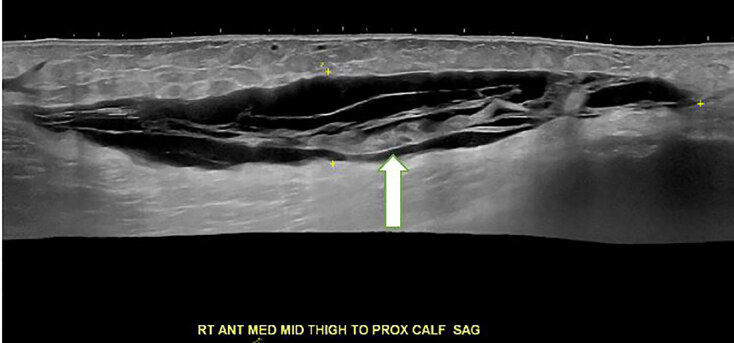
Ultrasound of the right lower extremity depicting the formation of a hypoechoic area between muscle and fascia (arrow).
